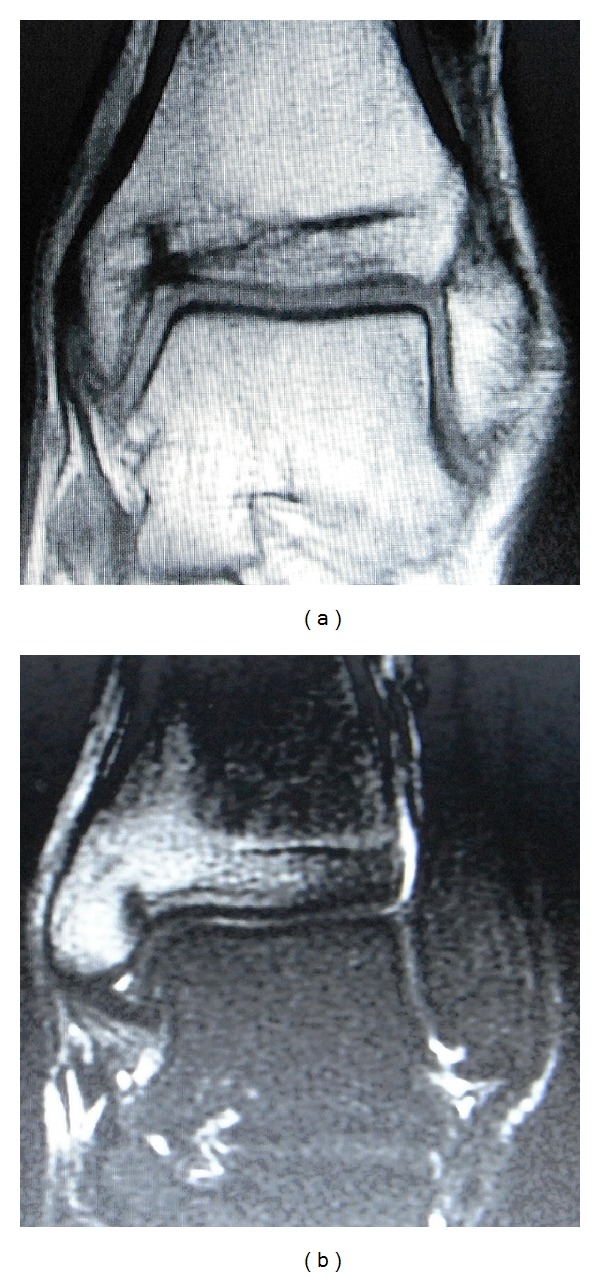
(a) T1-weighted MR image demonstrates a vertical linear lesion of low signal intensity originating from the superomedial corner of the ankle. (b) STIR (short T1 inversion recovery) image reveals bone marrow edema surrounding the linear lesion.
